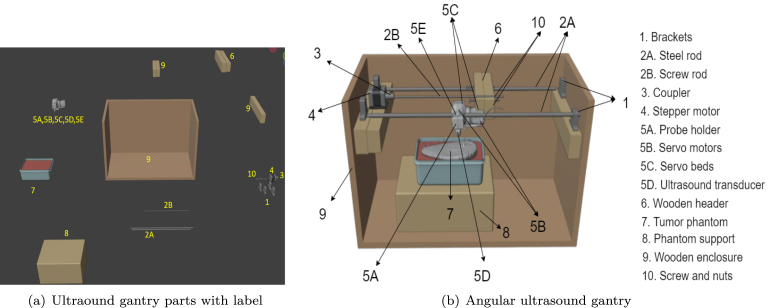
(a) shows the labeled components of the angular ultrasound gantry, and (b) shows the fully assembled angular ultrasound gantry with corresponding labels.
